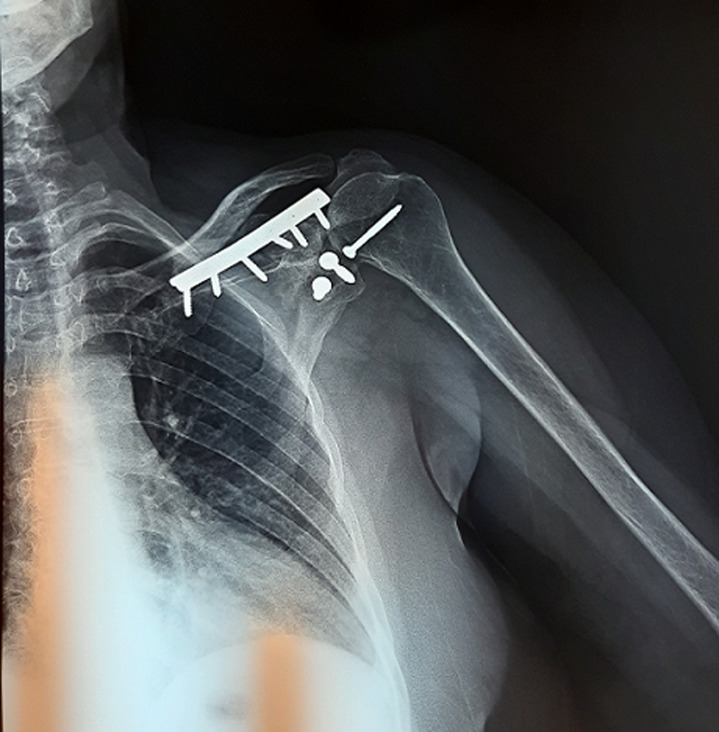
shoulder radiograph at 2 years showing no signs of osteoarthritis or osteonecrosis
